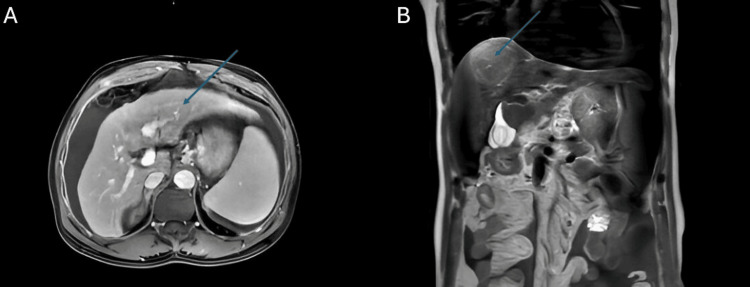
Magnetic resonance imaging of the liver with contrast Panel A: Axial T1-weighted post-contrast image demonstrates a large LI-RADS 5 lesion (arrow) in segment VIII of the right hepatic lobe, showing characteristic arterial phase hyperenhancement and washout, with evidence of extracapsular rupture into the subphrenic space. Panel B: Coronal T2-weighted image reveals the same lesion (arrow), adjacent to ascites. Additional subcentimeter nodules (not shown) elsewhere in the liver were categorized as LI-RADS 3 (probably benign) and LI-RADS 4 (suspicious, not definite for HCC). LI-RADS: Liver Imaging Reporting and Data System
